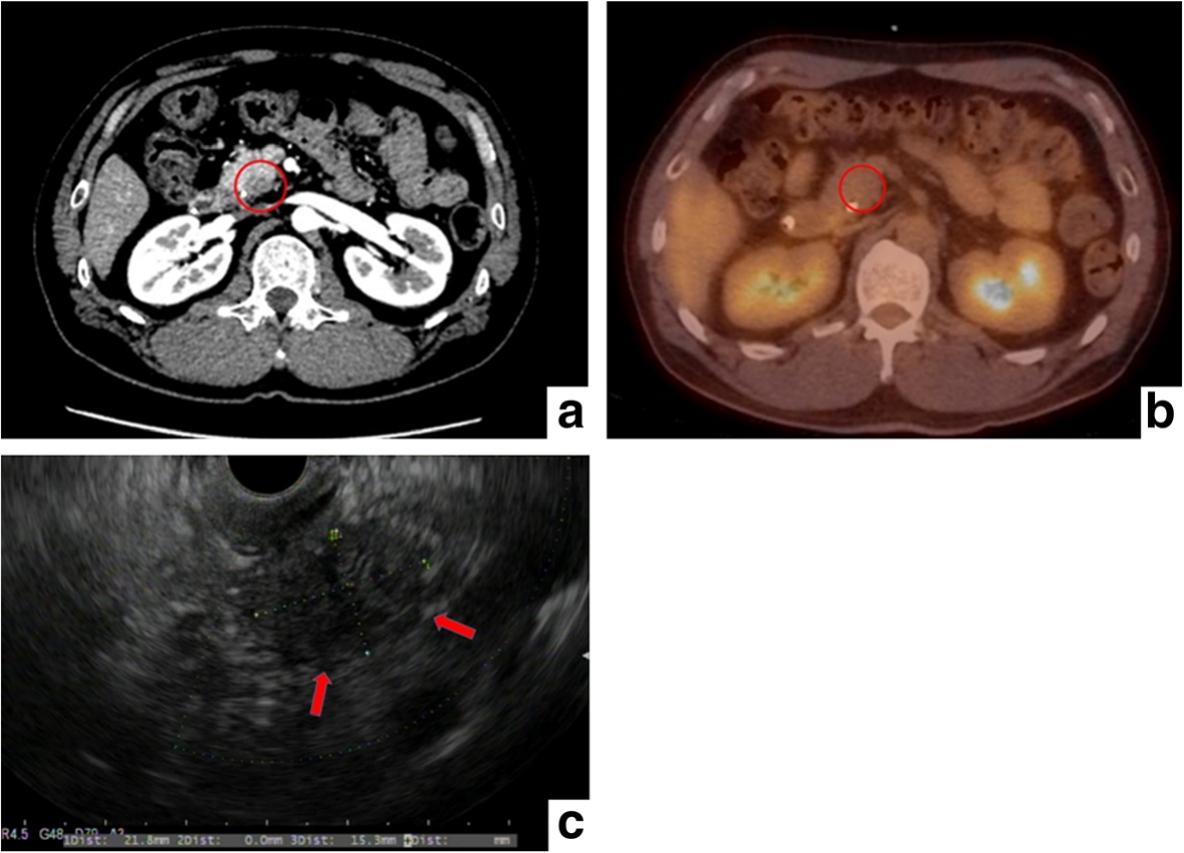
Radiological and endoscopic ultrasonographic findings for case 1. a CECT revealed a hypovascular tumor in the uncus of the pancreas (*red circle*). b PET-CT revealed that 18 F-FDG accumulation was within normal range. c EUS revealed a 22-mm hypoechoic lesion in the uncus of the pancreas (*red arrows*)
